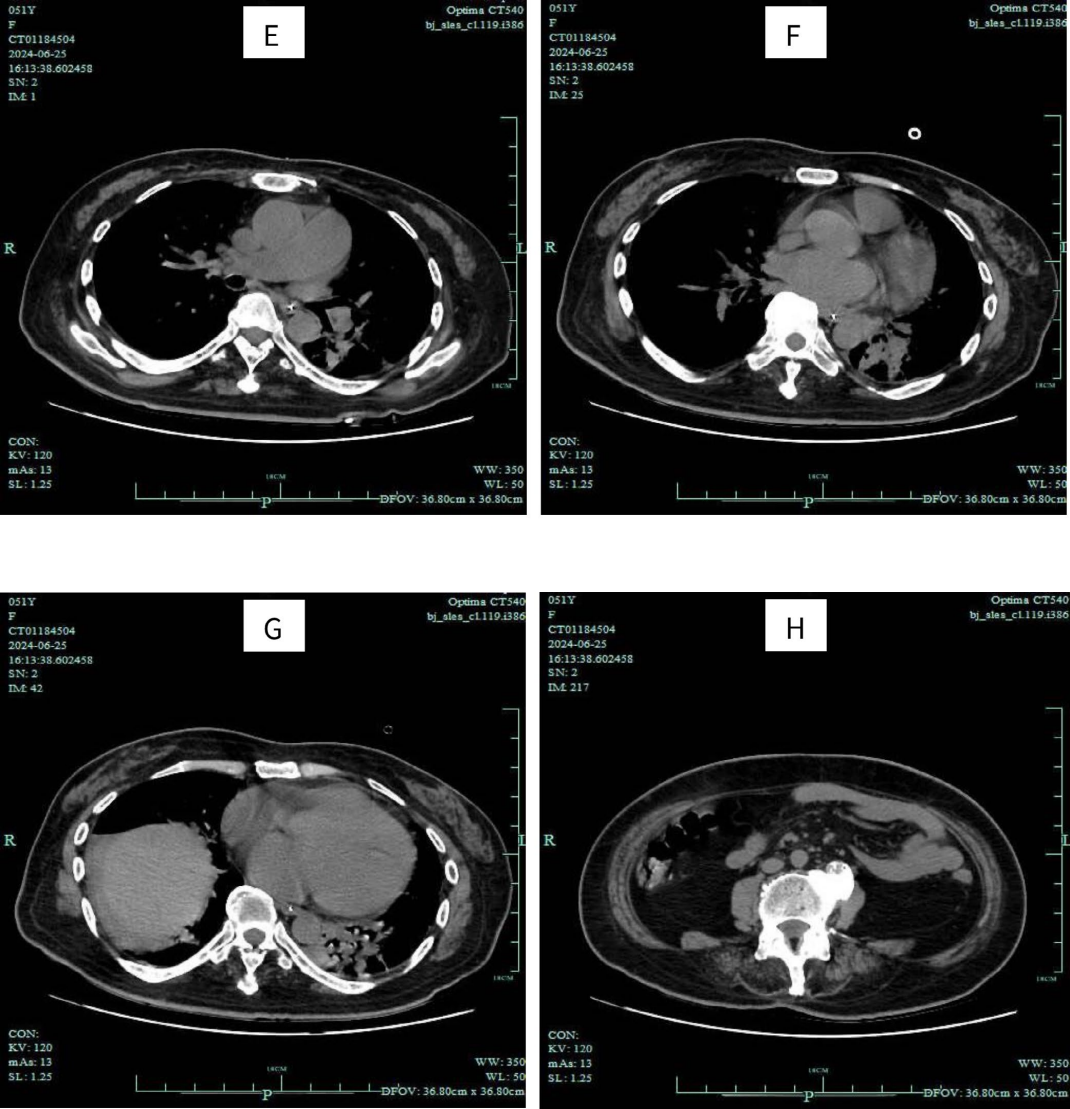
Results of noncontrast CT scan of the whole abdomen.
